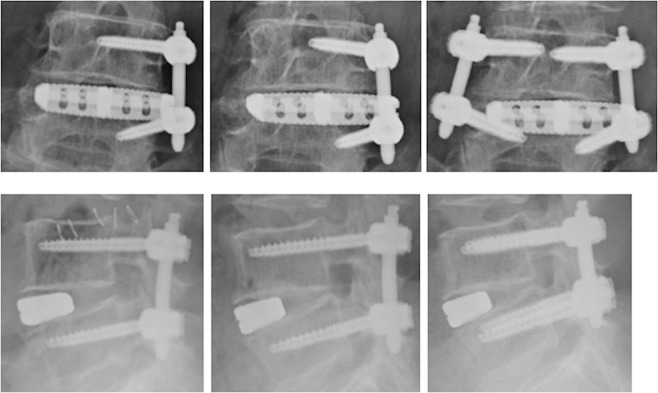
Radiographs of a 72-year-old woman showing after surgery at L4–5; LLIF with unilateral PPS. After 3 months, radiographs revealed loosening of the cage. After 18 months from re-operation, radiographs showed positions remained unchanged.
